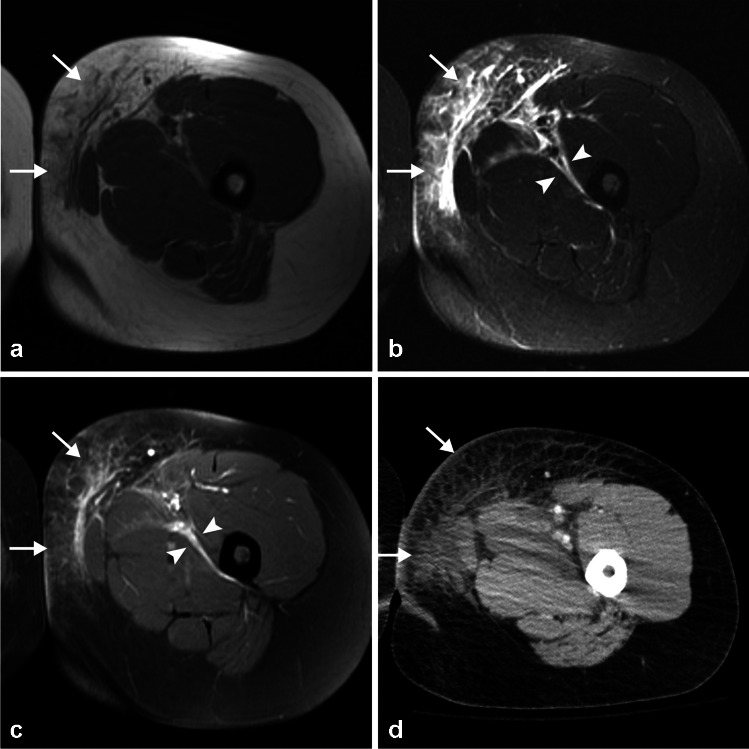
Twenty-two-year-old woman with left groin and thigh pain for 6 days. Axial T1 (a) and STIR (b) images show thickening and fluid along the deep peripheral fascia overlying the adductor and extensor compartments of the thigh (arrows) with extension along the deep intermuscular fascia between the two compartments and the muscles of the adductor compartment (arrowheads). T1 FS image following the administration of intravenous gadolinium-based contrast (c) shows enhancement of these fasciae. There is also edema and enhancement within the overlying subcutaneous tissues with thickening of the skin. Two days later, there was no improvement in patient’s condition and a CT scan with contrast (d) was performed which show similar findings. Necrotizing fasciitis was confirmed at surgery
